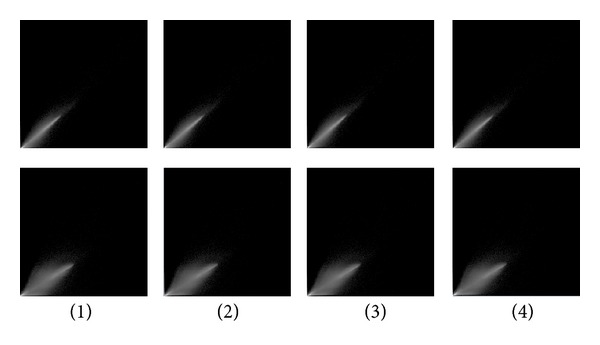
Joint histogram for registered 3D MRI volumes using an intensity-based cost function (CR (first column), LS (second column), NCC (third column), and NMI (fourth column)) are shown. The top row is generated from the images when registered using windowed Sinc interpolation, and the bottom row is generated from the images when registered using B-spline 3rd-order interpolation.
